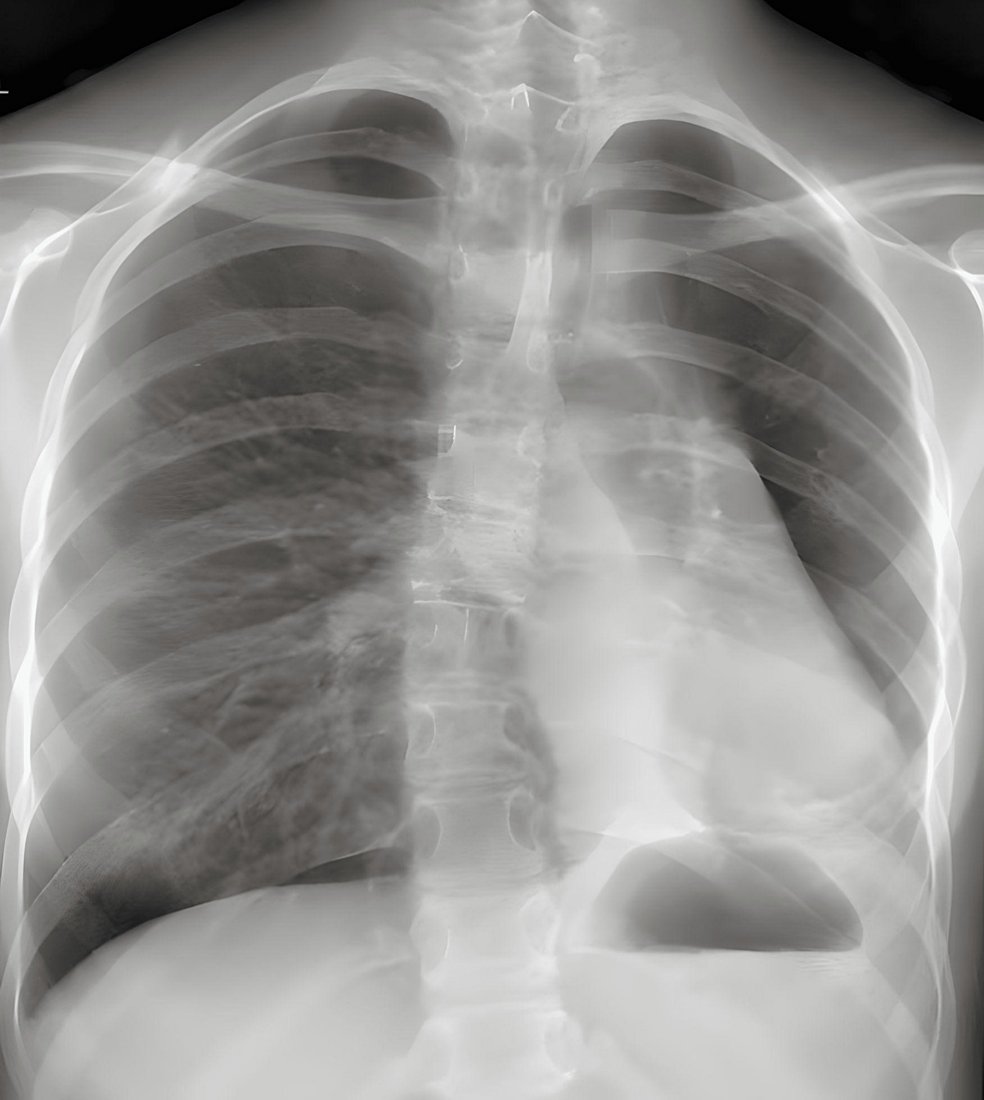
Chest X-ray showing hyperinflation of the right lung. Left retrocardiac consolidation or atelectasis. There is the elevation of the left hemidiaphragm. The cardiomediastinal silhouette is deviated to the left.
